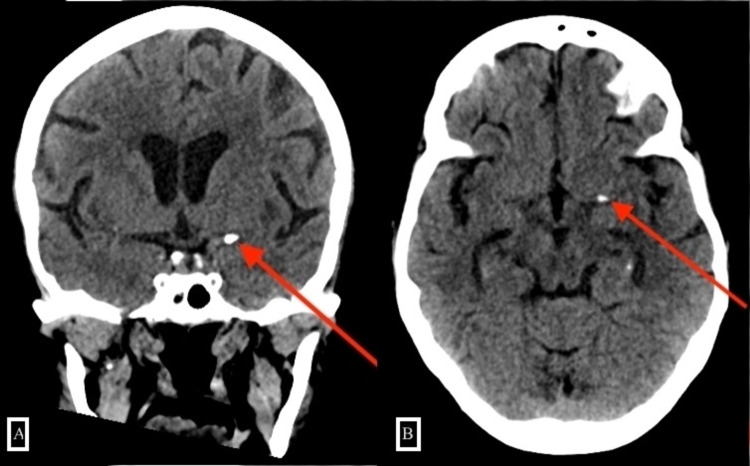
Nonenhanced CT of the head shows a calcified embolus within the left M1 segment of the MCA. MCA, middle cerebral artery
